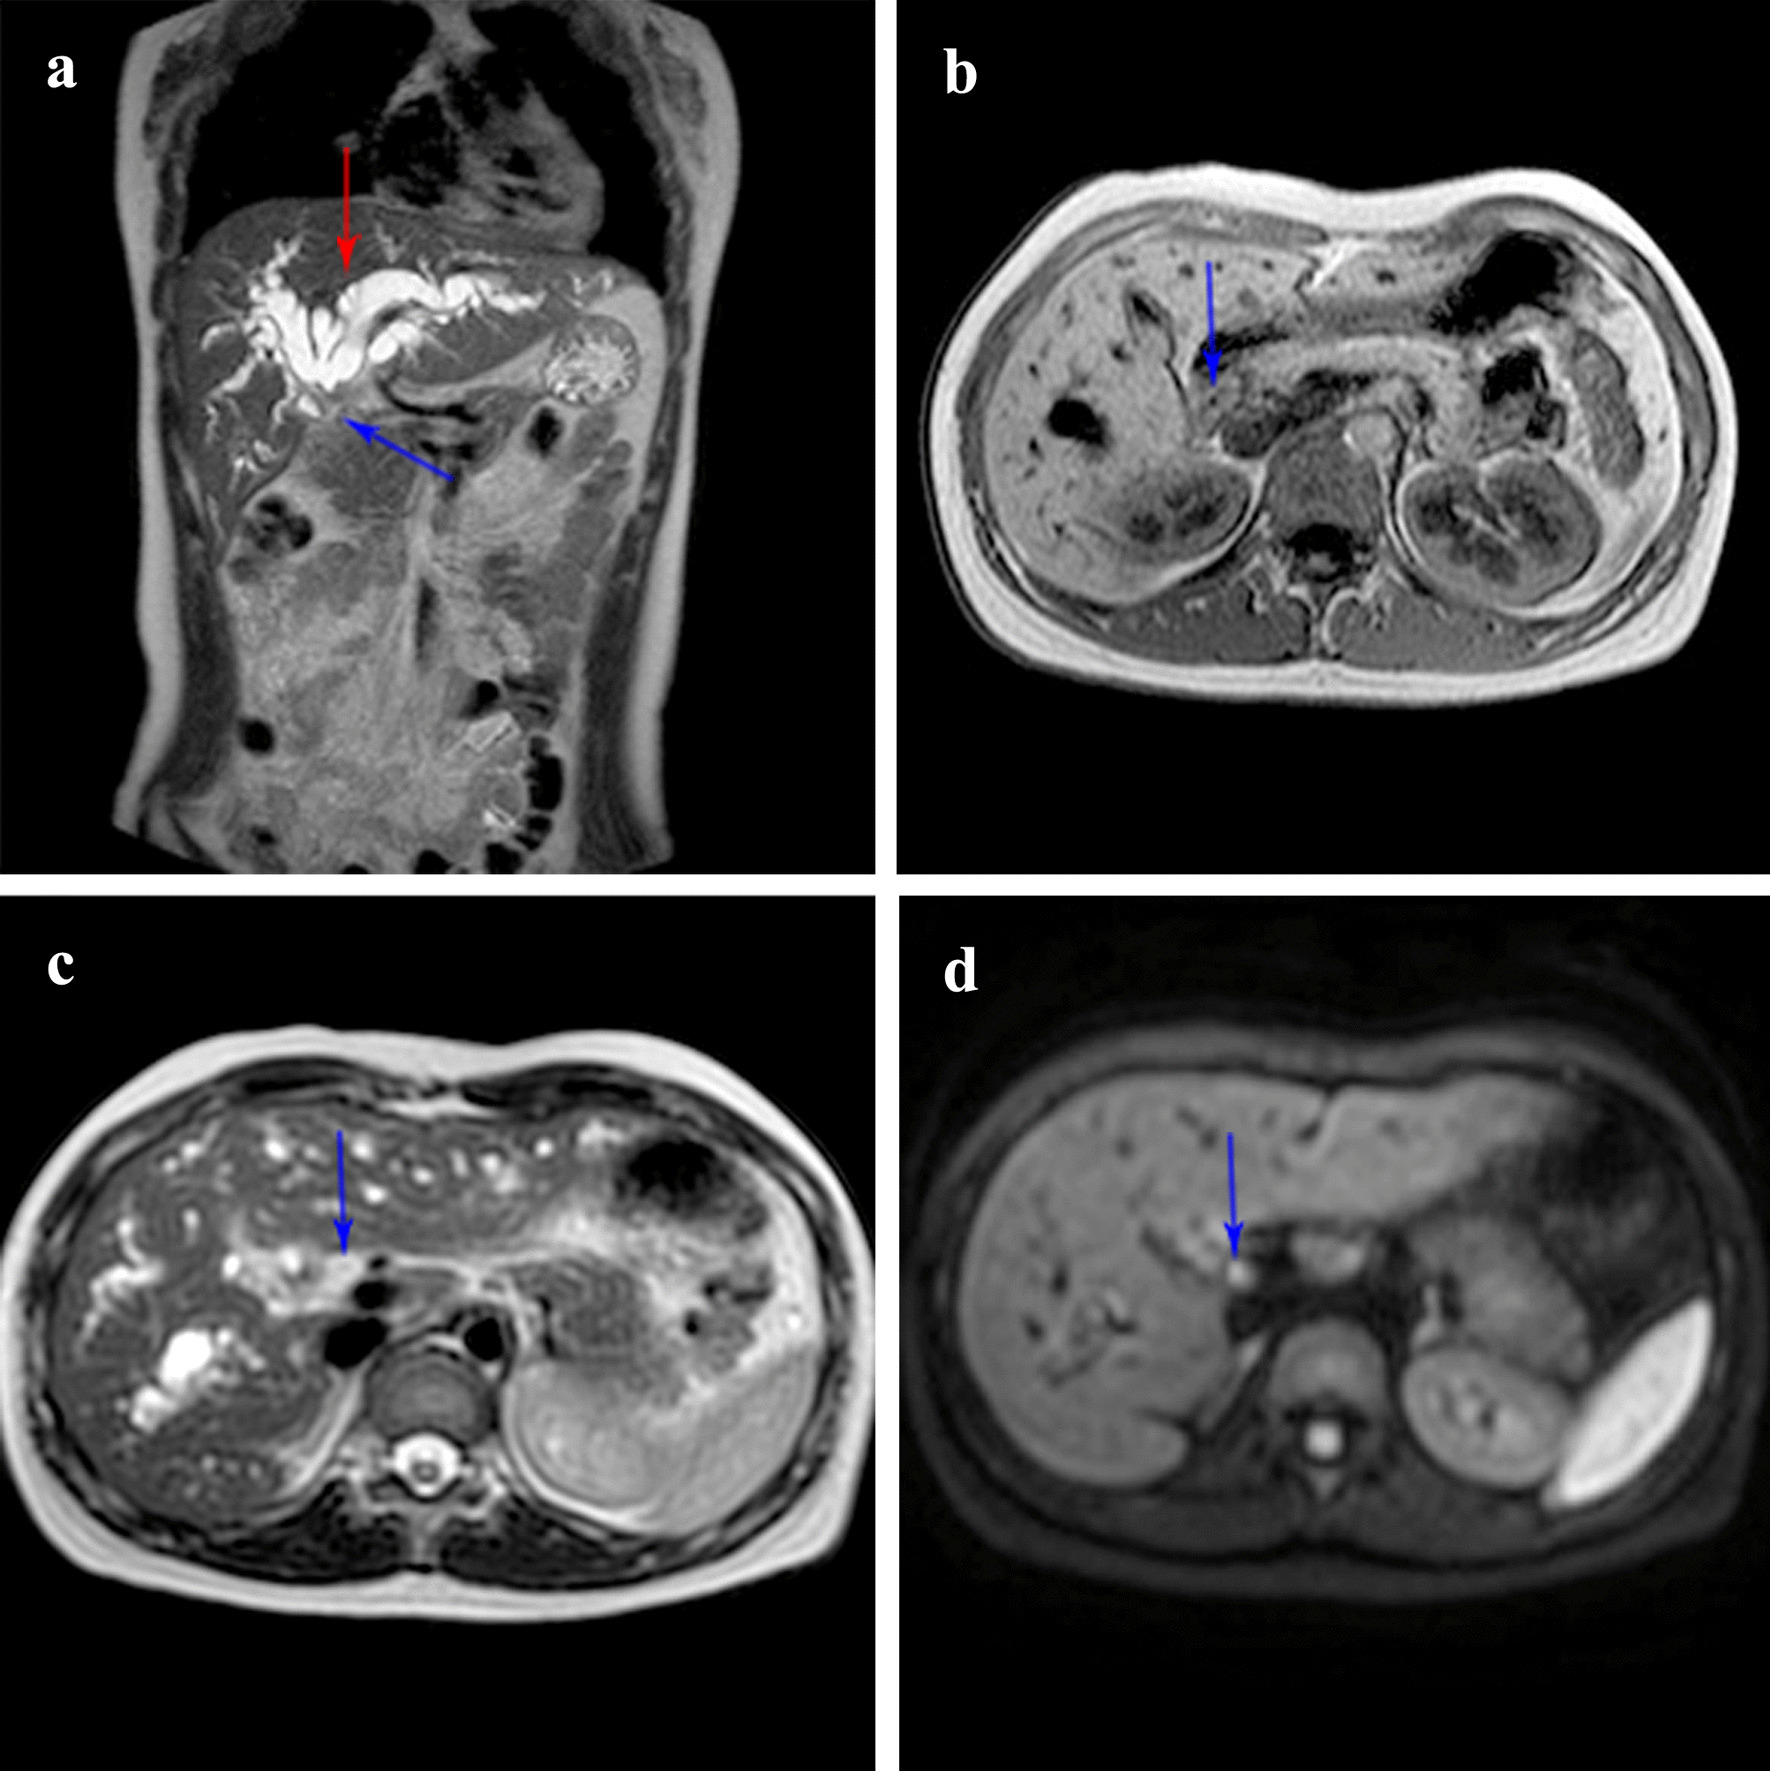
Preoperative abdominal MRI. a Magnetic resonance cholangiopancreatography (MRCP) showed that the tumor was located near the bifurcation of the hepatic duct (blue arrow) with diffuse intrahepatic bile duct dilation (red arrow). b T1-weighted image (T1WI) showed that the tumor had lower signal intensity (SI) than the hepatic parenchyma (blue arrow). c T2-weighted image (T2WI) showed that the tumor had higher SI than the hepatic parenchyma (blue arrow). d Diffusion-weighted image (DWI) showed the tumor had higher SI than the hepatic parenchyma (blue arrow)
